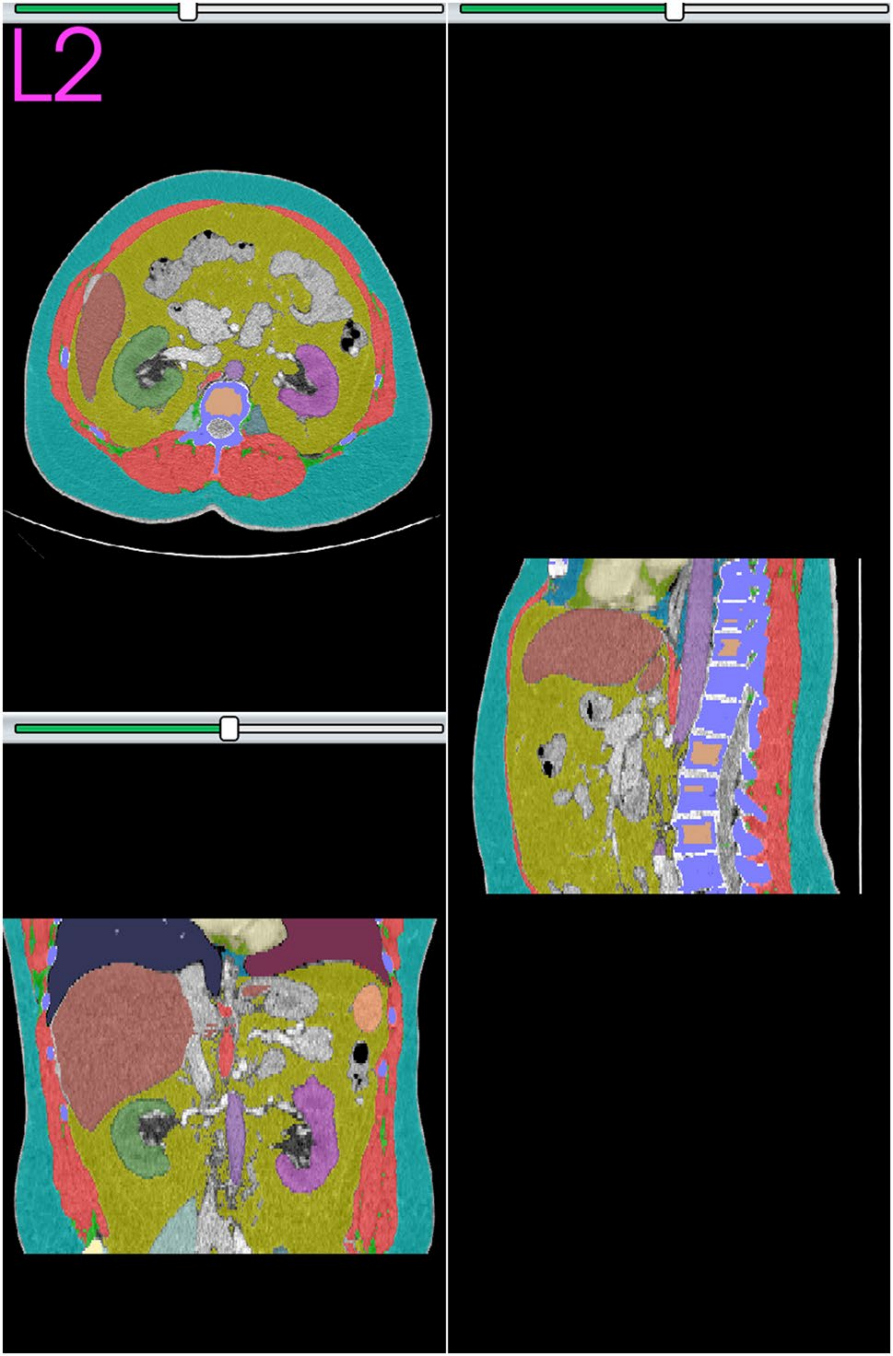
Axial CT scan image at L2 mid with automated body composition in a 53-year-old man with ACC.
